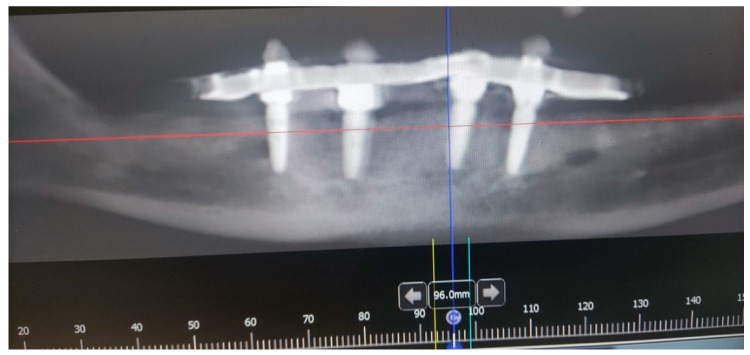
Orthopantomogram of the lower jaw of the patient.
